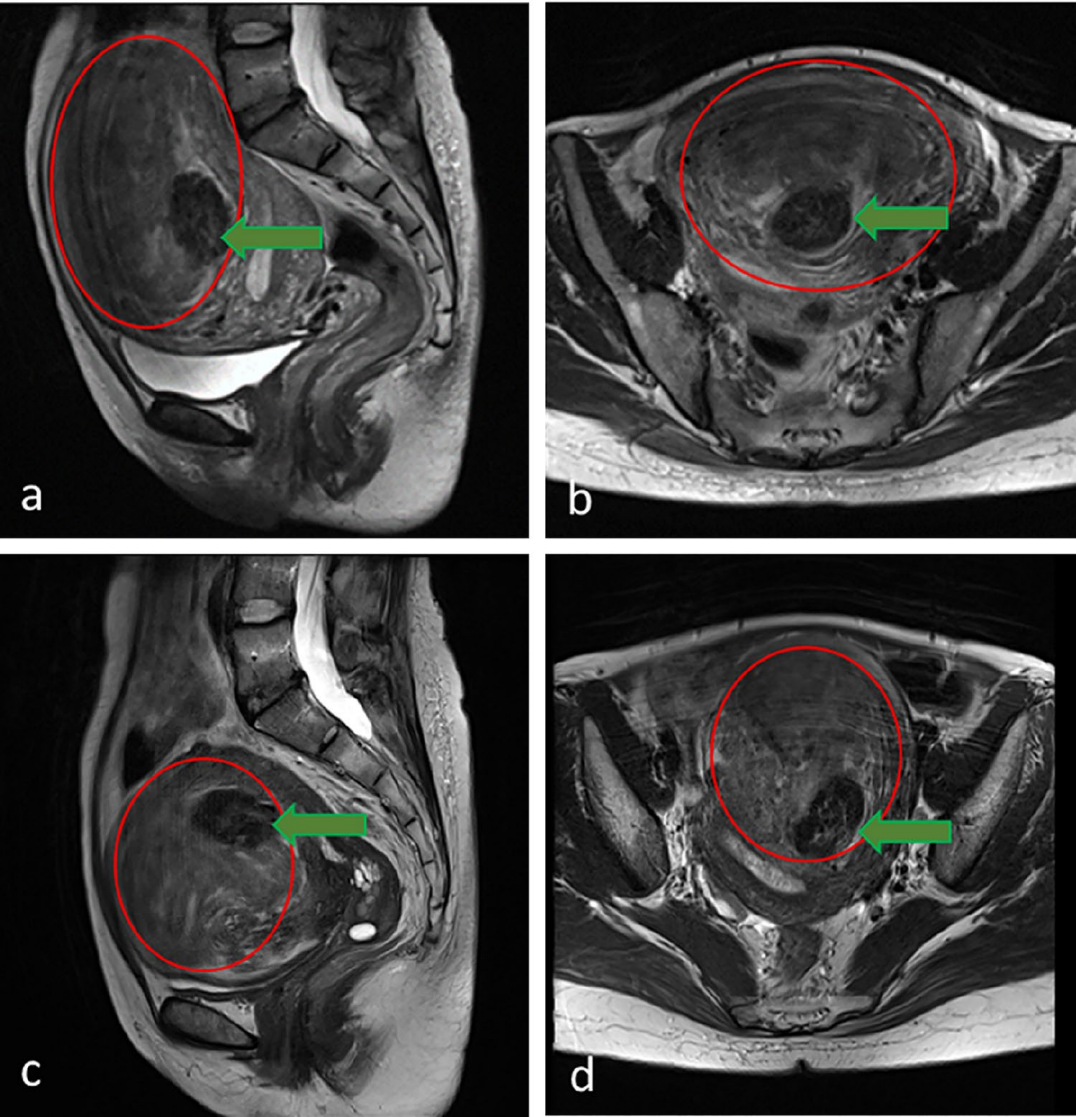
T2-weighted MR images of case no. 4, a 49-year-old woman with Funaki type 1 within the Funaki type 3 fibroid. Images include pretreatment (a, b) and post-treatment three-month follow-up (c, d). The pre-treated volume of fibroid was 525.6 cm^3^, and the post-treated volume was 216.2 cm^3^, with a shrinkage rate of 58.87%. Sagittal view: (a, c); axial view: (b, d). Red circles were the area of Funaki type 3 uterine fibroid; green arrows were the area of Funaki type 1 uterine fibroid
